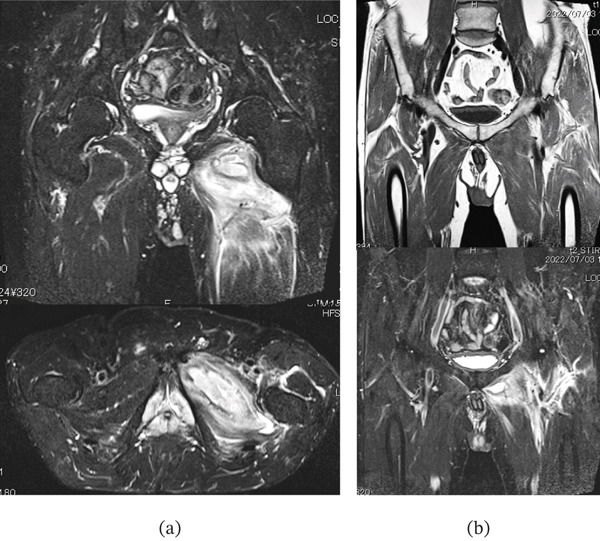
Case 1. (a) Preoperative coronal and axial hip MRI with short T1 inversion recovery (STIR) images and (b) coronal MRI with T1‐weighted imaging and STIR images of the lower lumbar spine and the hip.
